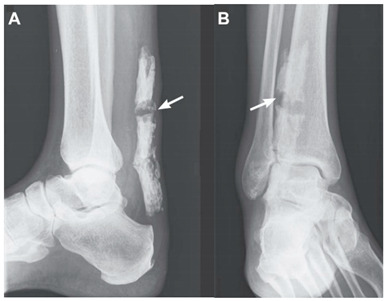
Lin et al., 2012 ^b^ [34]	A 70-year-old woman. This image submission includes two radiographs of a 70-year-old woman (Image C). The patient presented with a sudden stabbing ankle pain. She denied any history of trauma or surgery but did report frequent local injections of her right ankle for pain and soreness. Radiographs demonstrate complete rupture of the calcified Achilles Tendon and a 3 cm separation shown in the first radiograph. Direct suture repair was performed and the follow-up radiograph revealed minimal gap in the tendon (Image D).
